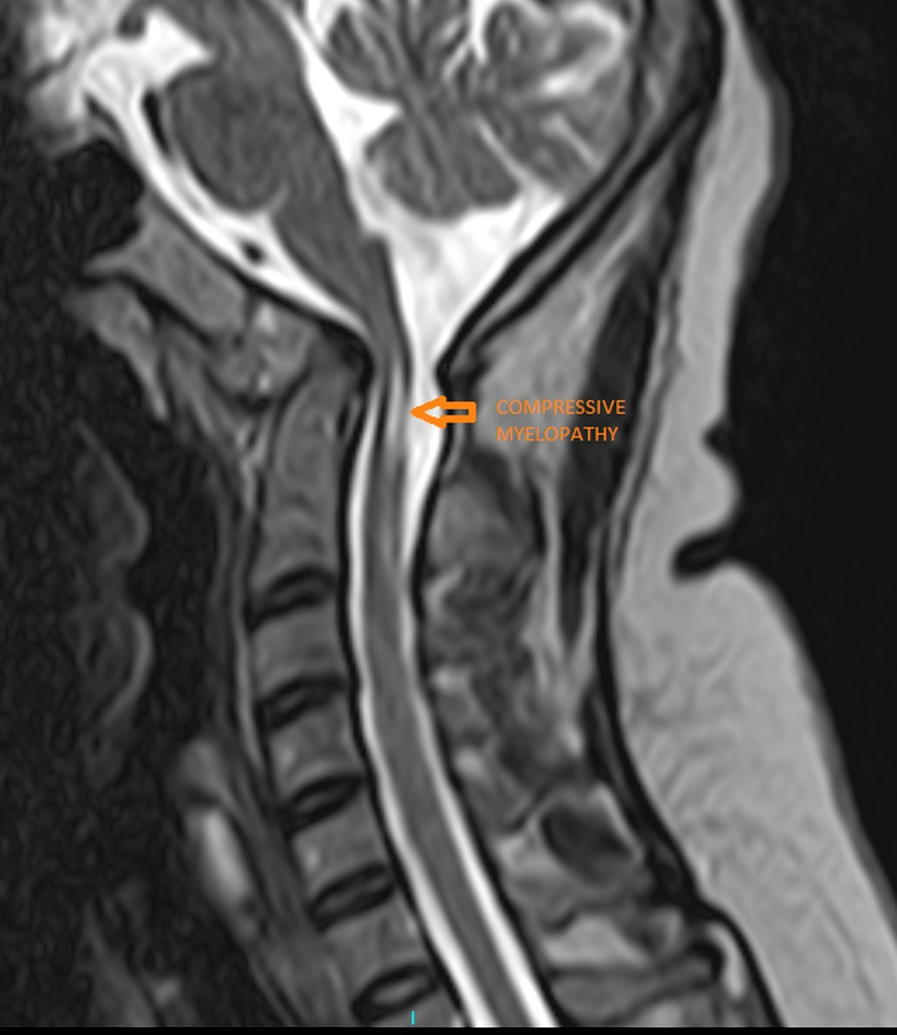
Sagittal T2-weighted magnetic resonance imaging showing compressive myelopathy
